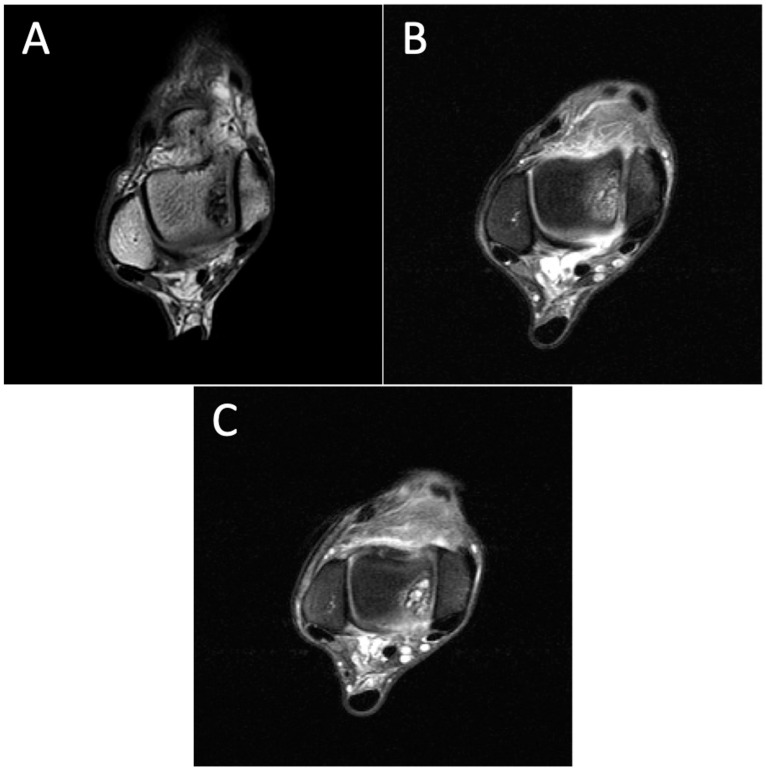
Degenerative progression of the right OLT on axial MRIs from 2011 to 2021. The timeline of OLT progression includes MRI scans from 2011 (A), 2015 (B), and 2021 (C).
